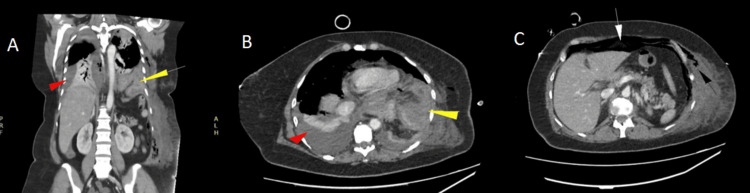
Contrast-enhanced CT images A) Coronal slice showing herniated stomach (yellow arrow) and pleural effusion (red arrow); B) Axial slice showing herniated stomach (yellow arrow) and pleural effusion (red arrow); C) Axial slice showing pneumoperitoneum (white arrow) and subcutaneous emphysema (black arrow). CT, computed tomography
